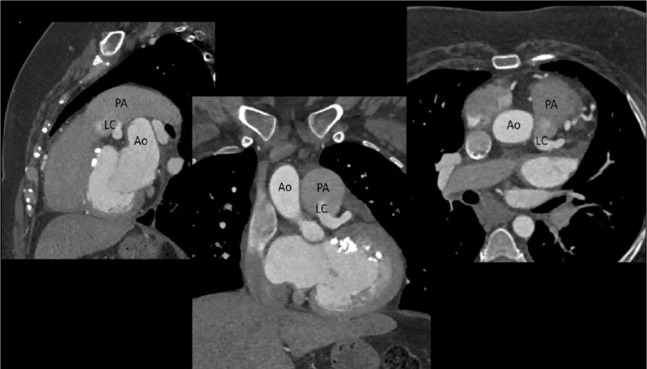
Sagittal, coronal, and transverse CT slices showing the origin of the left coronary artery (LC) from the pulmonary artery (PA). The aorta (Ao) is shown in perspective.
